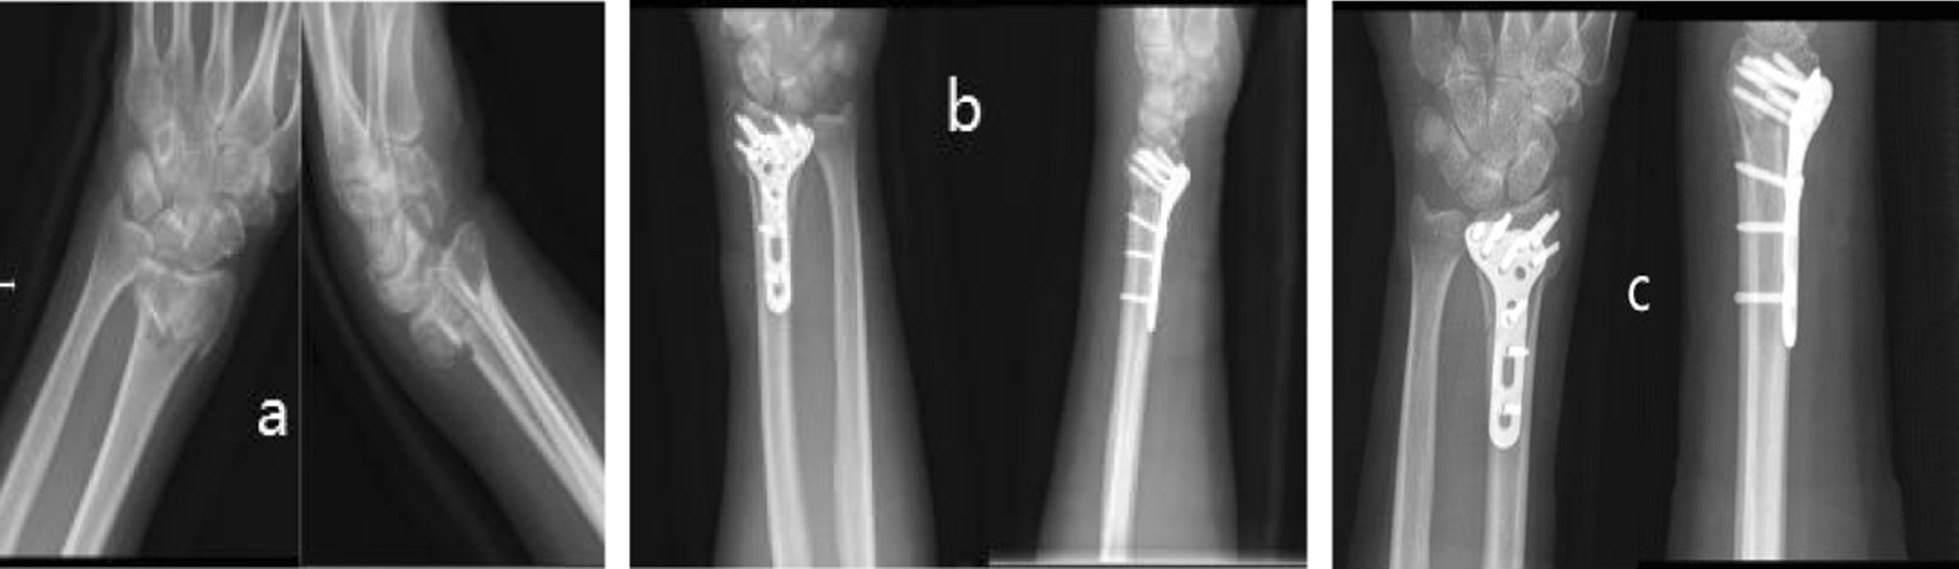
X-ray observations of Case 1. a Shows a preoperative X-ray showing a fracture of the right distal radius; b Shows the X-ray on the next day of surgery, showing the exact reduction and fixation of the fracture; c Shows the X-ray at 6 weeks after surgery with blurred fracture lines
